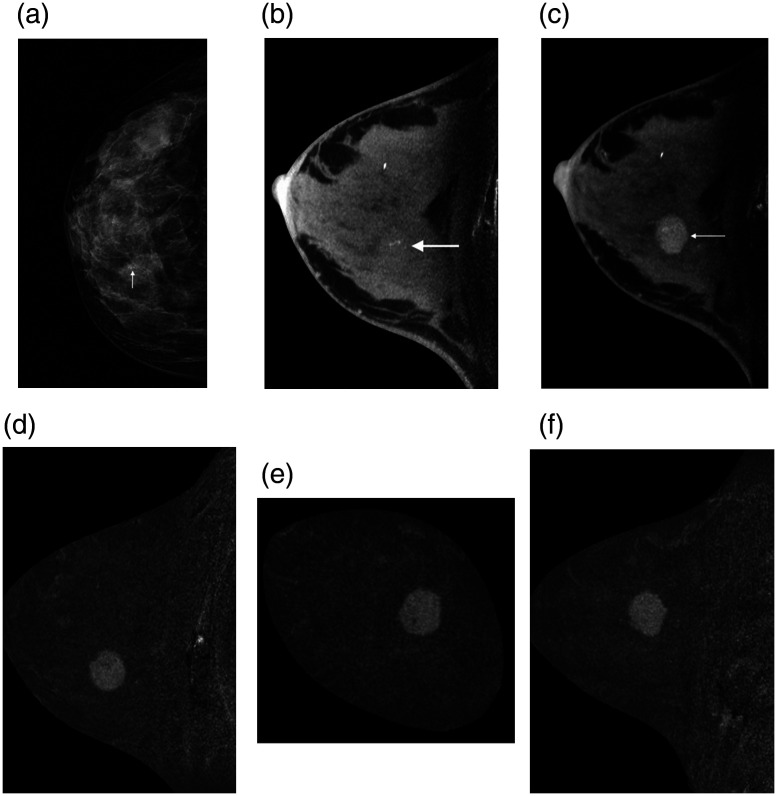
(a) Craniocaudal (CC) view mammogram showing suspicious clustered microcalcifications (arrow) in the inner part of the right breast. The density of the tumor is superimposed by normal breast tissue. (b) The microcalcification cluster is visible in the dedicated noncontrast cone-beam breast CT (arrow). (c) Addition of contrast for contrast-enhanced cone-beam breast CT shows a clearly delineated, intensely homogeneous contrast enhanced tumor mass (arrow). (d) Axial, (e) coronal, and (f) sagittal views of the subtraction images show the enhancement in the tumor mass, which was determined to be a ductal invasive carcinoma. Reprinted from Wienbeck et al.^129^ with permission from Elsevier.
